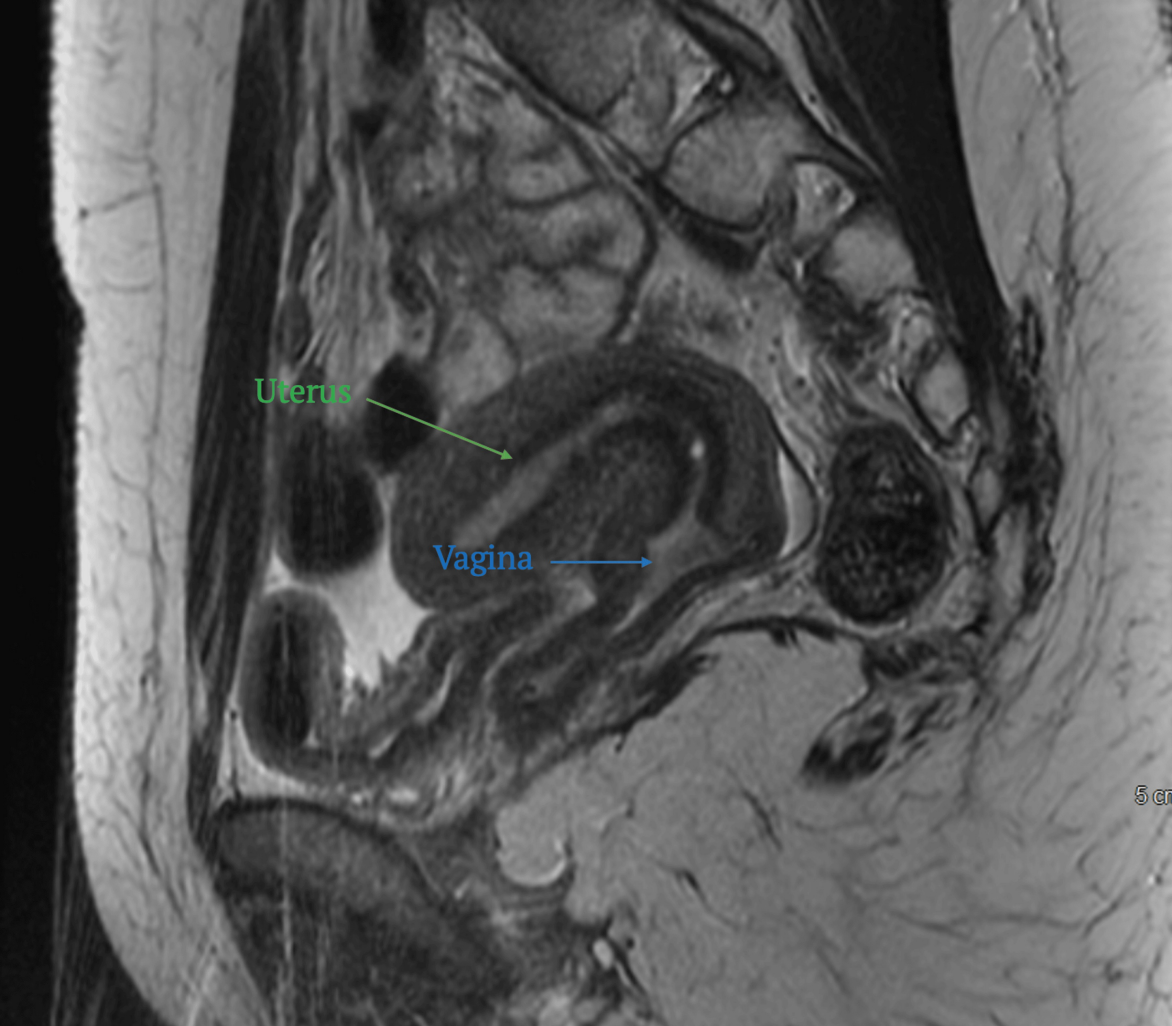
Postoperative MRI revealed reduced distension of the vagina with residual hematocolpos and air-fluid levels. A single, non-septate vagina was observed (blue arrow). The uterus appeared normal, with an arcuate shape and smooth indentation of the fundal endometrial canal measuring 0.5 cm (green arrow).
